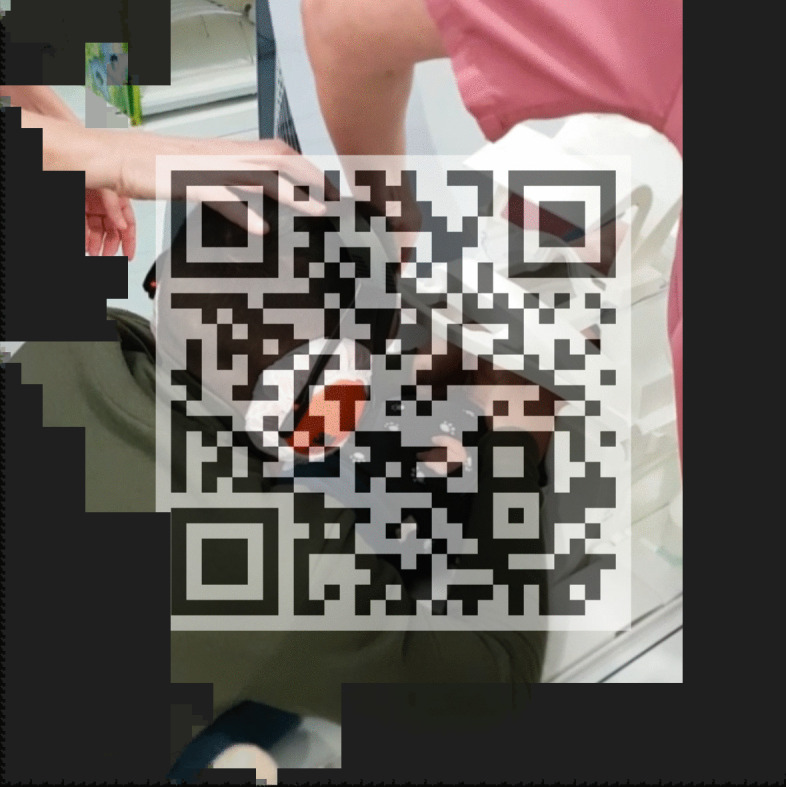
Video 1 Video of a real-time MRI in a non-sedated infant in our department. The father is placed together with the child in the scanner bore of the MRI. He gently supports the patient’s head with the flat of his hands. Remaining movements of the child do not interfere with imaging because of the ultrafast acquisition time down to 20 ms per image
